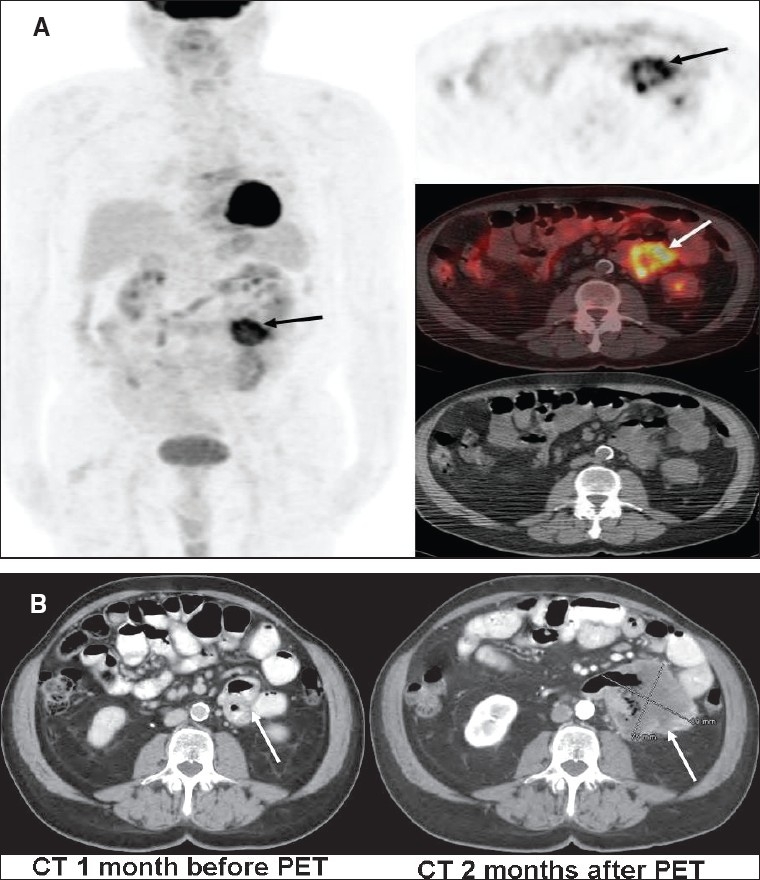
Axial PET (top right), fused PET/CT (middle right) and CT scan (bottom right) images at the level of the mid-abdomen, with an MIP image (on left), from a PET study (A) of a patient with treated melanoma. The images show an intense gut-associated focus in the left abdomen (arrows), strongly suspicious of recurrence. However, a structural lesion that could be biopsied was evident only 2 months later (B) on a CT scan (right image). No structural lesion was evident even retrospectively on a prior CT scan (left image)
